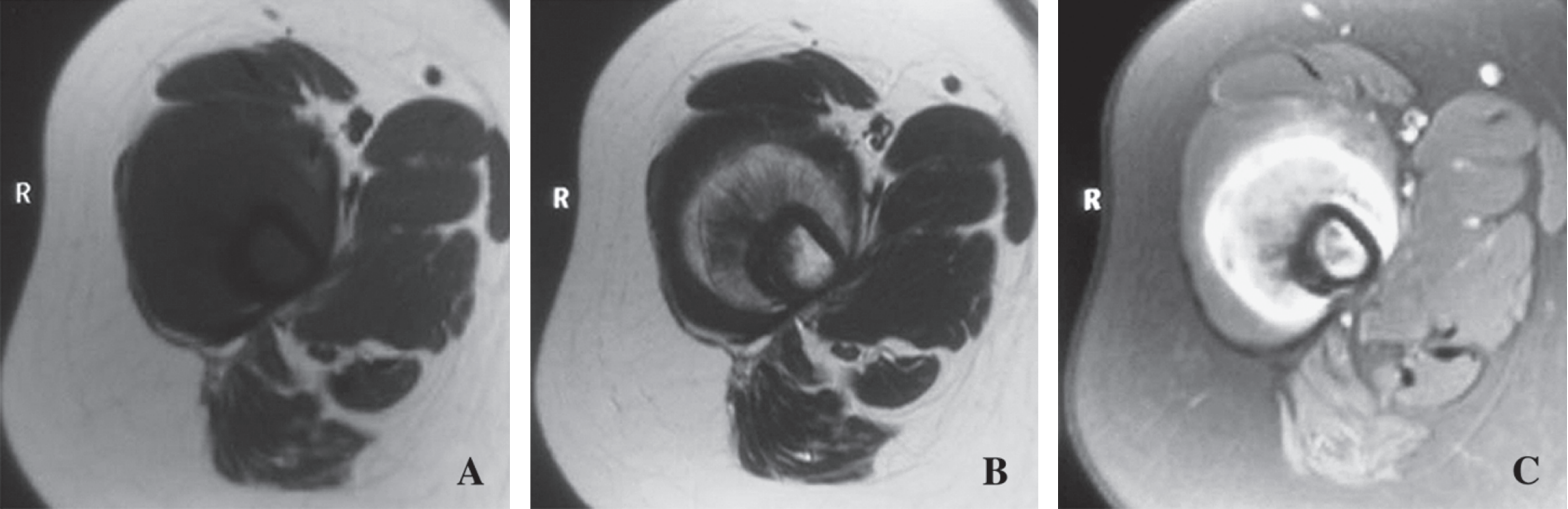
Case1. (A) MRI revealed an isointense lesion within the quadriceps femoris muscle on T1-weighed images. (B) T2-weighed images showed a heterogenous hyperintense lesion. (C) The lesion was enhanced heterogeneously on fat-suppressed T1-weighed images after gadolinium injection.
